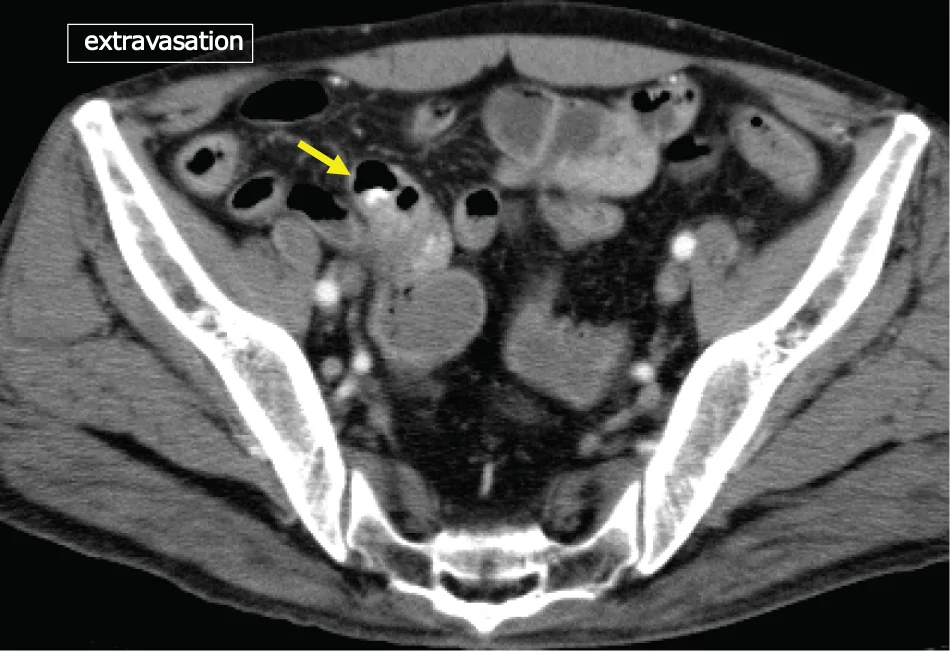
Contrast-enhanced CT findings. Contrast-enhanced CT demonstrated a hyperdense area in the colon and extravasation of contrast in the ileum, indicating active gastrointestinal bleeding without a clear mass lesion.
